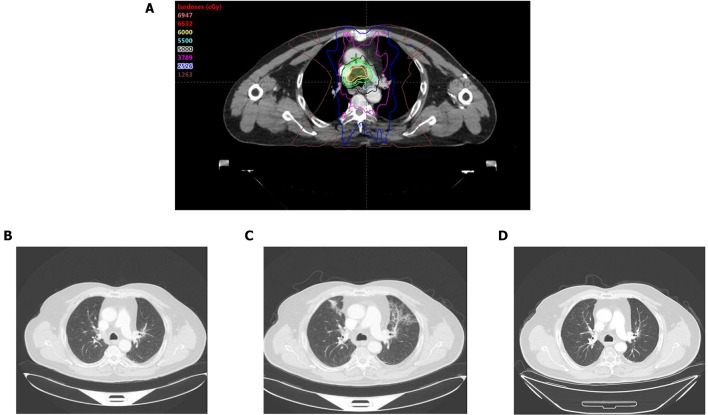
(A) Radiation field. (B) Before administration of Camrelizumab, CT scan showed no pneumonitis manifestation. (C) After administration of Camrelizumab, CT scan revealed patchy consolidation and ground-glass opacities localized within the previously irradiated area. (D) Reexamination of CT showed significant improvement in pneumonitis 2 weeks after administration of prednisolone.
